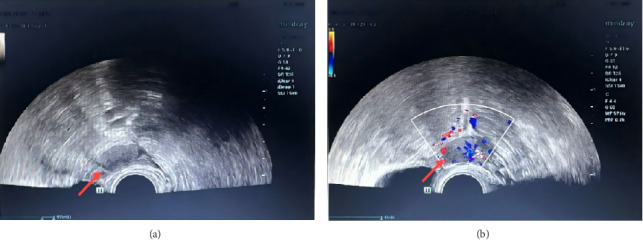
The ultrasound shows a solid, hypoechoic mass that is intimately attached to the outer border of the right ovary and has abundant blood flow signals.
